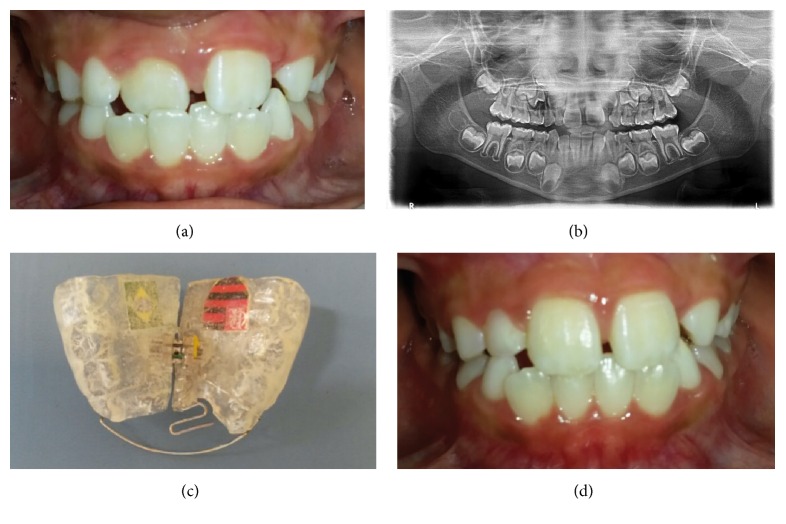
Case  1. (a) Anterior dental crossbite in the upper right central incisor. (b) Panoramic radiograph showing no abnormality. (c) Removable orthodontic appliance with a protrusion spring for incisor in anterior crossbite. (d) Anterior crossbite correction after 3 months.
